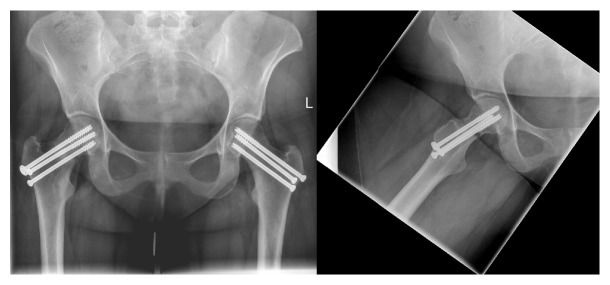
Radiographic evidence of femoral neck fracture nonunion, taken at 10 months postoperatively.
